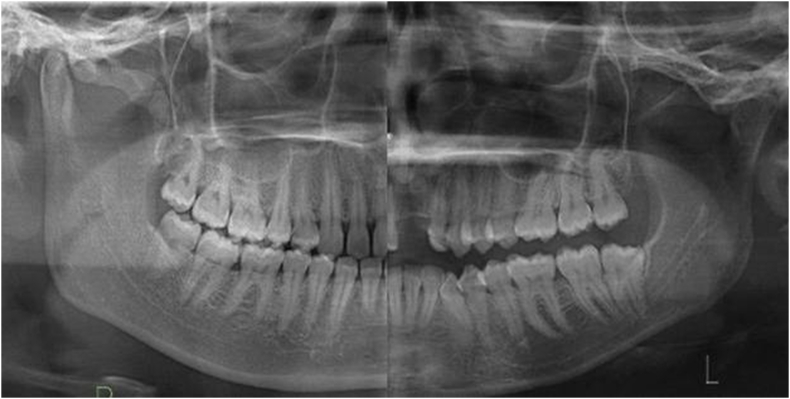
A combination of radiographic image showing two different presentations of root to mandible ratio. Left image shows a ratio of about 50 % while a higher ratio is shown in the right image.
